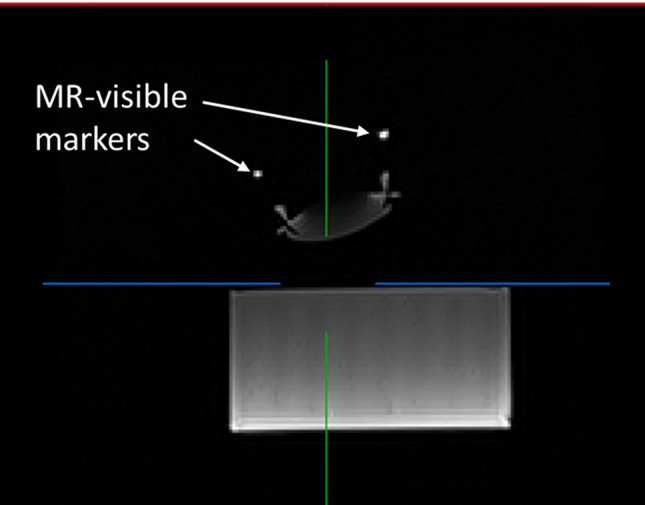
T1-weighted MR image used for the estimation of the transducer position and orientation in the MR scanner.
